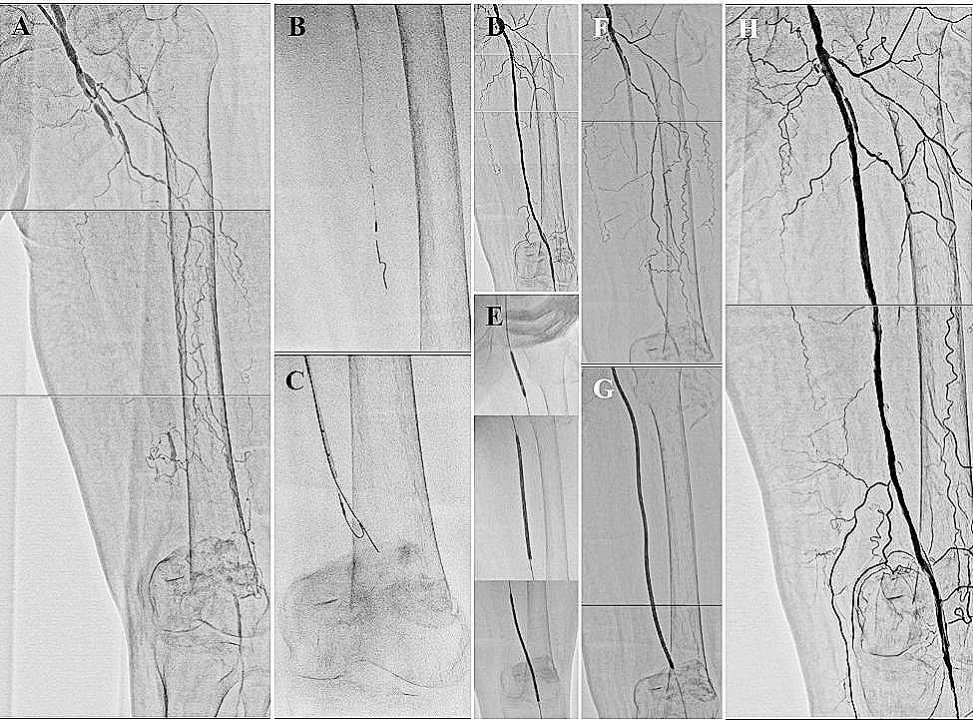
a Initial angiography showing a long chronic occlusion from proximal superficial femoral artery (SFA) to mid-popliteal artery. b Guidewire manipulation with intravascular ultrasound (IVUS) guidance. c Parallel wiring to track the intra-plaque area d Sufficient vessel dilation with favorable blood flow after prolonged balloon inflation. e Inflation of three drug-coated balloons (DCB) throughout the SFA. f Angiography post DCB inflation demonstrating blood flow stasis at the mid segment of the SFA. g Prolonged balloon inflation for another 5 min. h Final angiography showing the recovery of favorable blood flow
